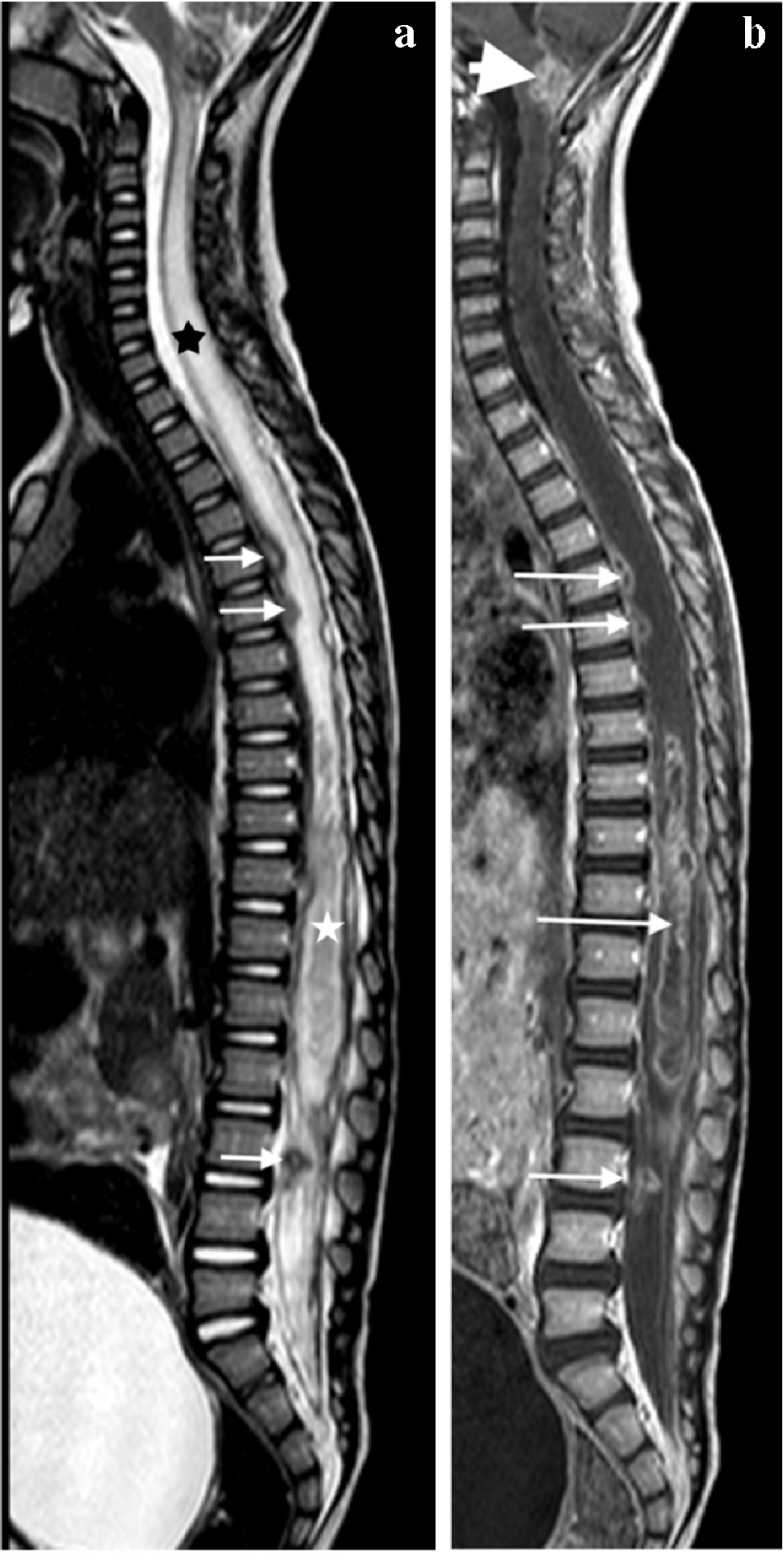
Sagittal magnetic resonance images in a 3-year-old girl with tuberculous meningitis and paraplegia. a T2 image demonstrates an irregular hypointense intramedullary lesion from T7 (*black asterisk*) to the conus (*white*
